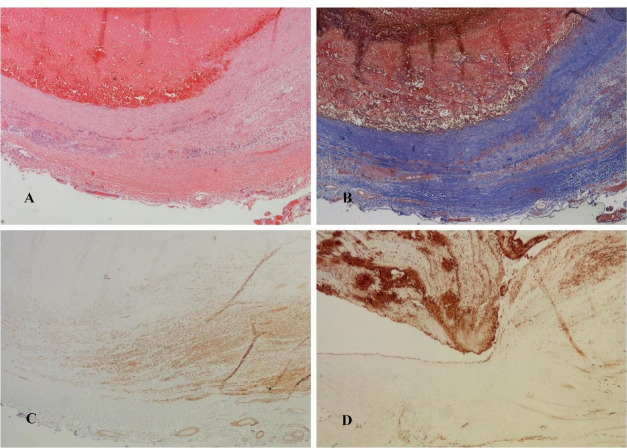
One month afterwards, at the follow-up visit, the patient denied having symptoms of hand ischemia and duplex ultrasound examination showed adequate patency of the radial artery.
